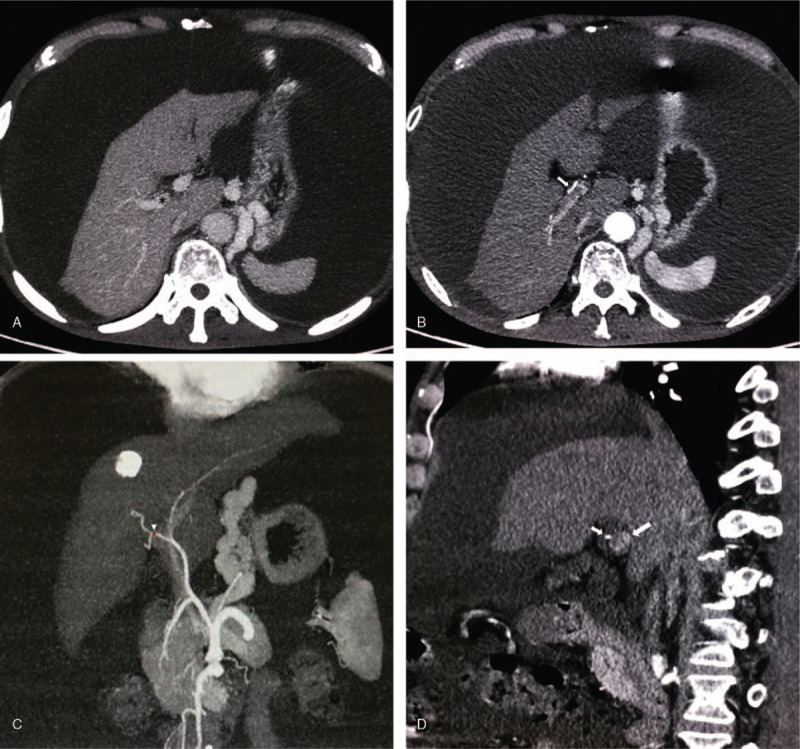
A. On the portal phase of the CT, the target entry zone of portal vein (∗) was determined. B. IHAL point (white arrow) ventral to the scheduled entry zone of the portal vein. C. A MIP image showed hepatic artery branch with the localization point on it (white arrow head). D. A MIP image showed the IHAL point (small arrow) and entry area of the portal vein (big arrow). IHAL = intrahepatic arterial localizer, MIP = maximum intensity projection.
